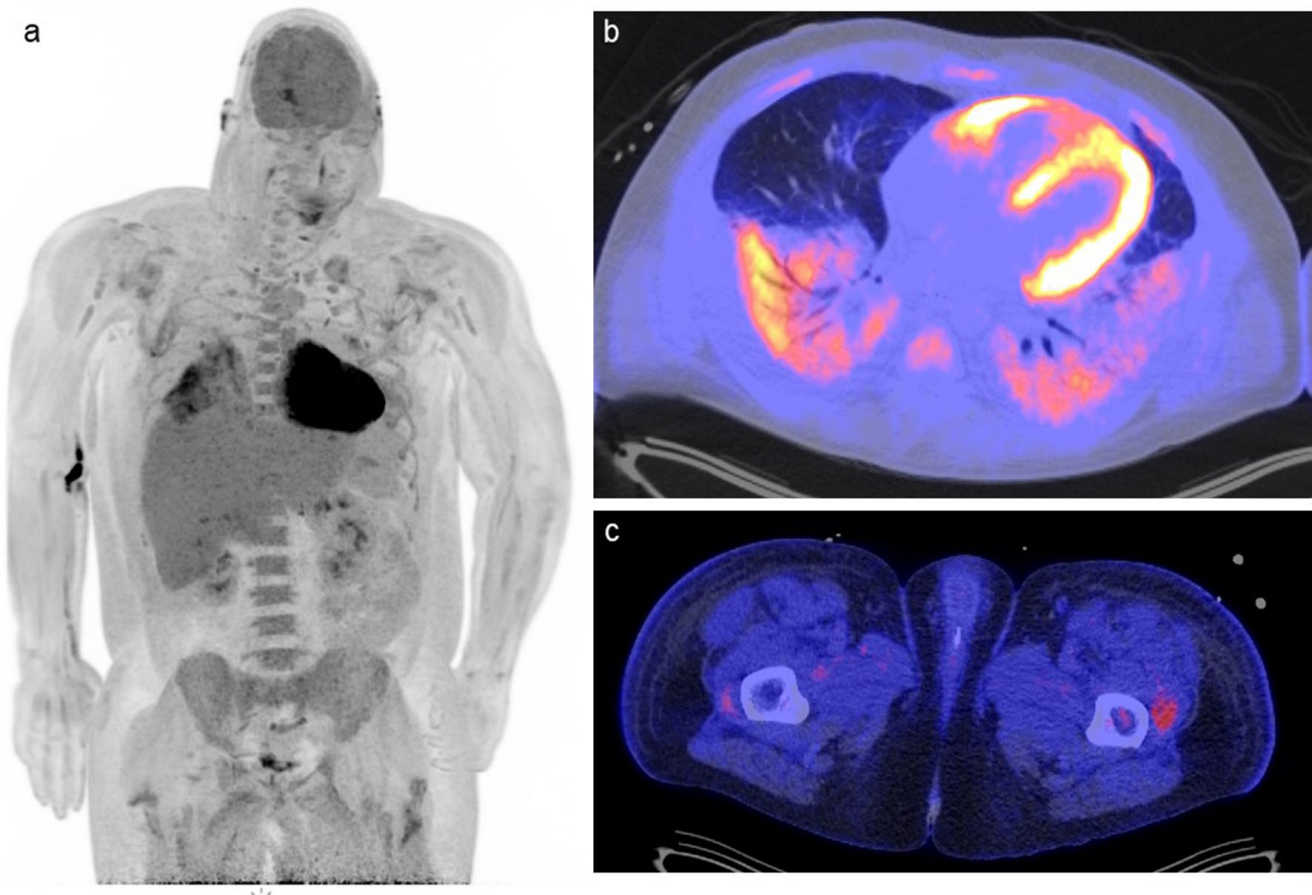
[^18^F]FDG-PET/CT of patient 3. A 27-year-old male was intubated 10 days after the onset of symptoms of COVID-19. Mechanical ventilation was partially combined with extracorporeal membrane oxygenation due to worsening of the patient's respiratory condition. When the patient’s condition deteriorated again, a second treatment with extracorporeal membrane oxygenation was not possible due to inflammation. An [^18^F]FDG-PET/CT was performed to identify a potential focus of infection. However, while the patient tested negative for SARS-CoV-2, no clear focus was identified on the PET/CT, transesophageal echo, or bronchoalveolar lavage. The patient was therefore treated with high-dose steroids, leading to a slow recovery. The patient was ventilated for over 2 months in total. a Maximum intensity projection. Physiological uptake in the liver. Atypical low intensity in the kidneys due to kidney failure. Reduced uptake in the brain due to sedation. There is no suppression of the heart, most likely due to short and insufficient fasting time. b Transaxial pulmonary fused PET/CT image with peripheral inferior atelectasis and consolidations on CT with [^18^F]FDG uptake. CT in lung setting c Transaxial fusion PET/CT of the hip with some slightly elevated uptake in the lateral muscle of the left hip. CT in soft tissue setting. *SUV-bw threshold of 5.00 α* = *50%*
